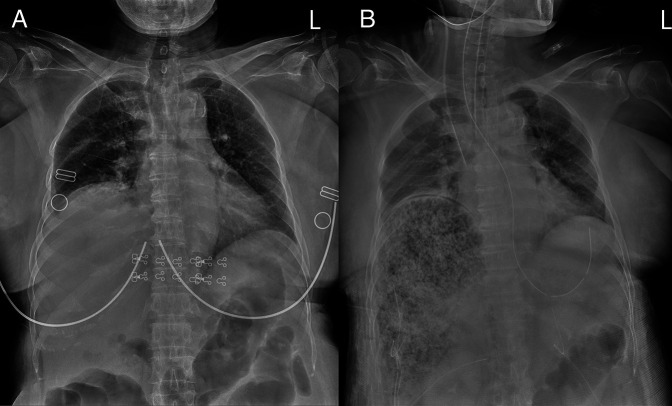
(A) Initial radiography demonstrated no prominent evidence of ongoing liver illness but showed a marked elevation of right-side diaphragm and ileus. (B) Two hours later, radiography showed gas-containing liver, with clinical rapid deterioration.
